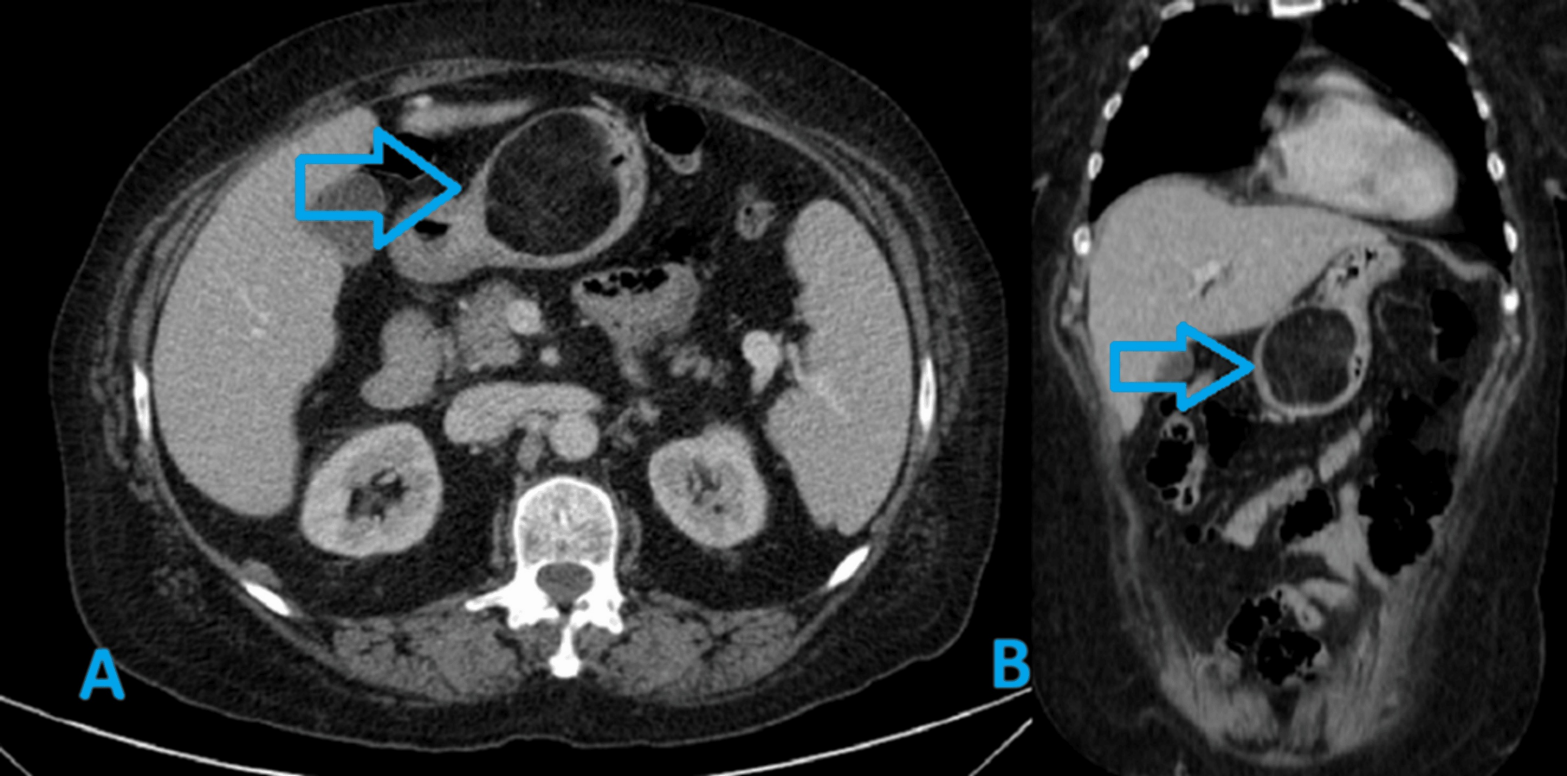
CT scan showing a lipomatous gastric lesion Axial (A) and coronal (B) views demonstrating a lipomatous gastric lesion (blue arrow) measuring approximately 56 mm originating from the greater curvature of the antrum, with attenuation value of -80 Hounsfield units (HU).
